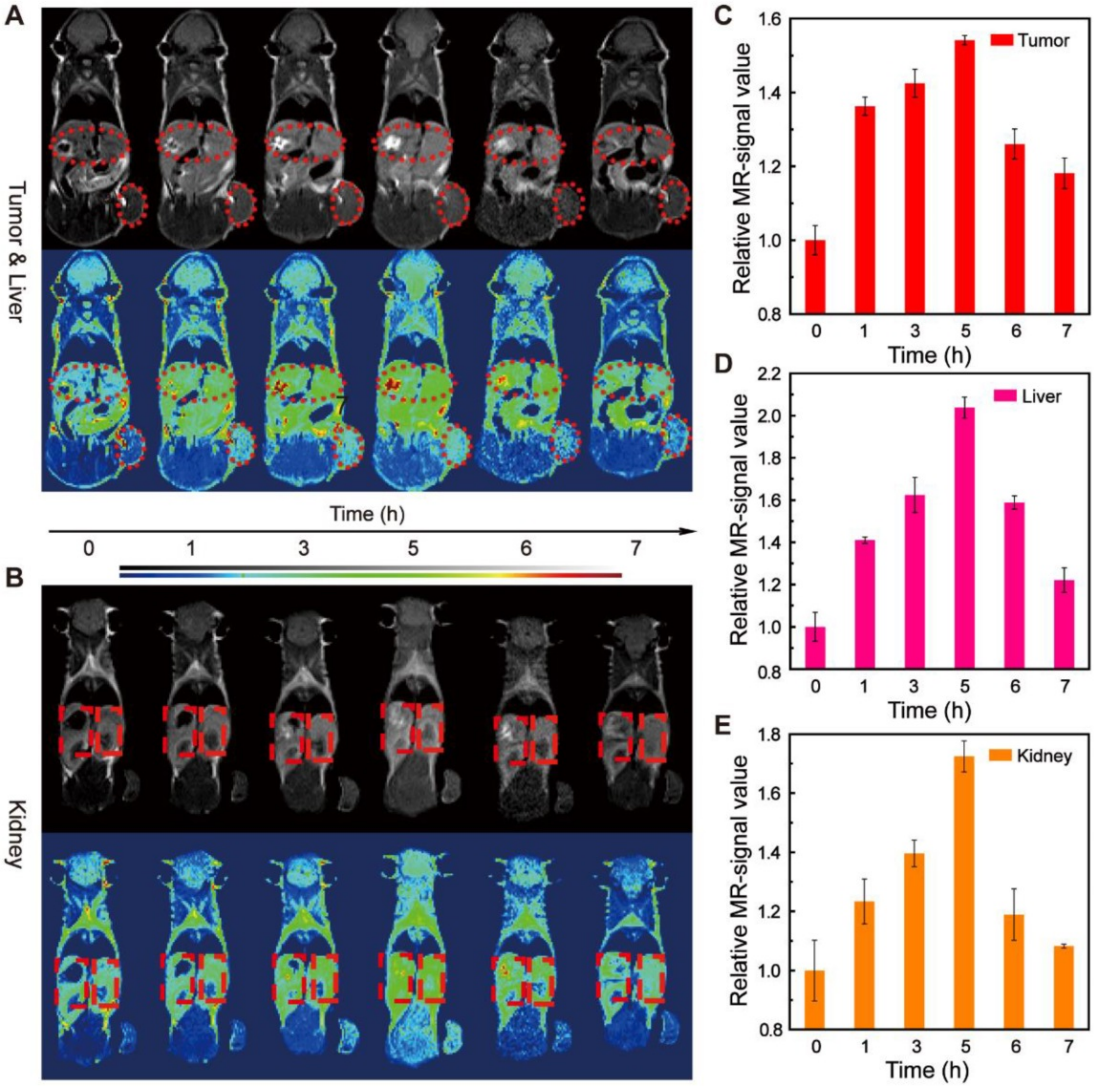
MRI images of the EA-Fe@BSA NPs *in vivo*. (A) MRI images of the tumor (indicated with small ellipses) and liver (indicated with large ellipses). (B) MRI images of the kidney (indicated with rectangles). (C-E) Corresponding MRI signal intensities for the images shown in (A, B).
